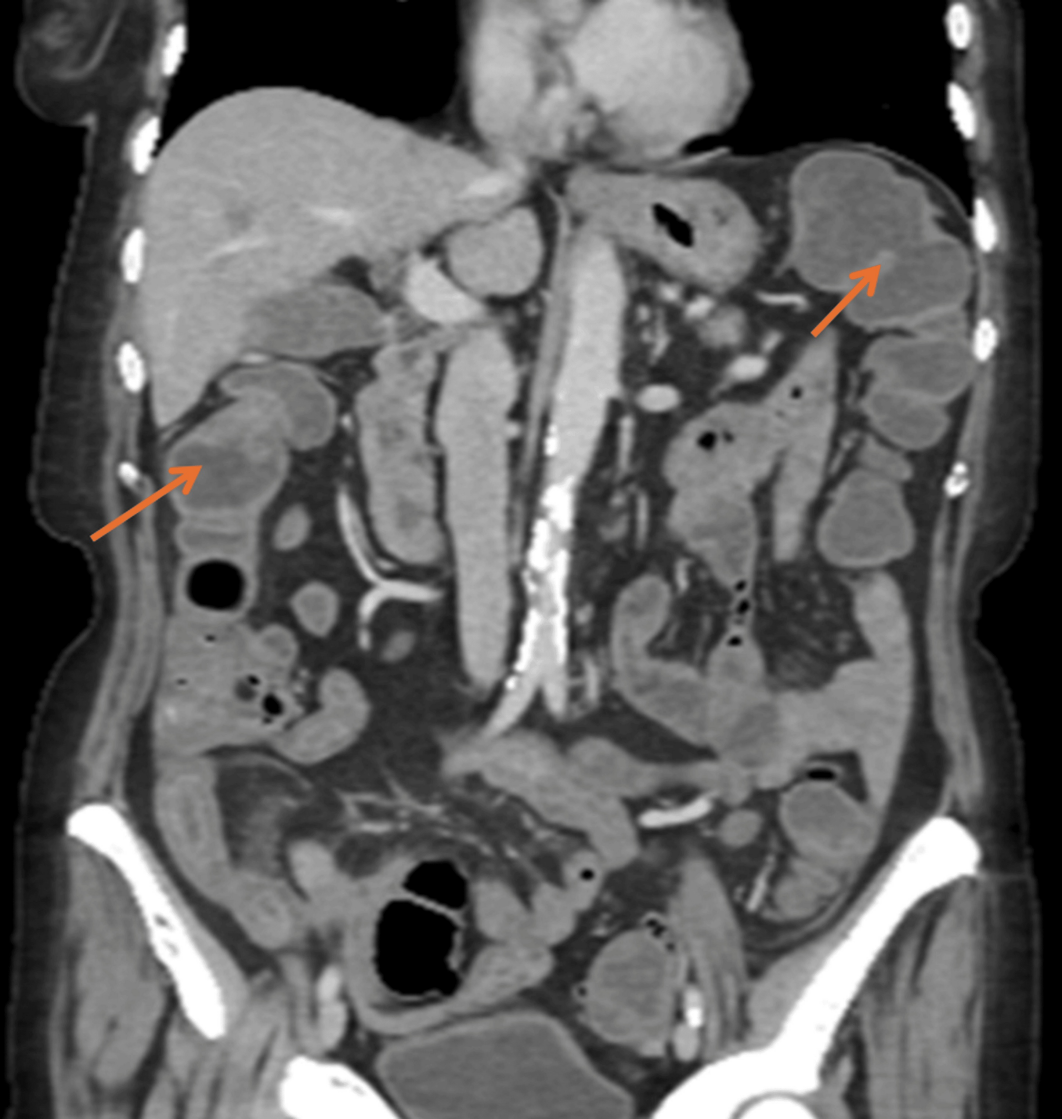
Coronal CT abdomen showing a long segment of mural thickening in the distal ileum (arrows).
